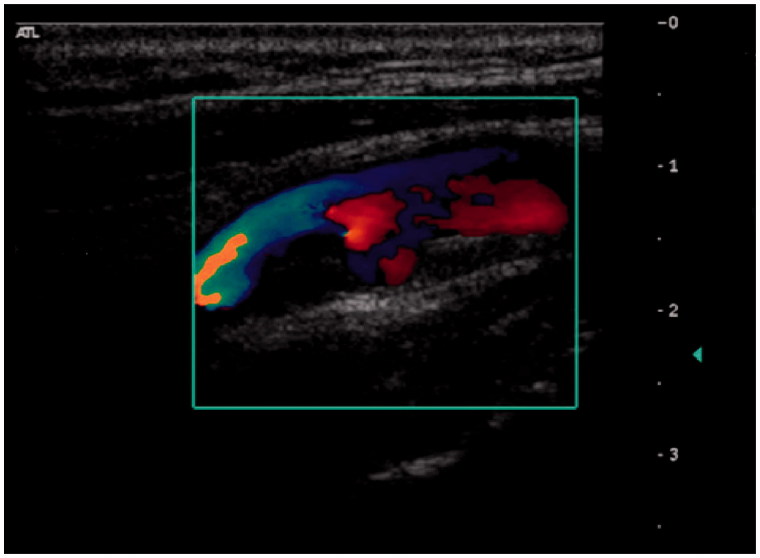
Carotid artery with stenosis and mosaic pattern on colour Doppler.
